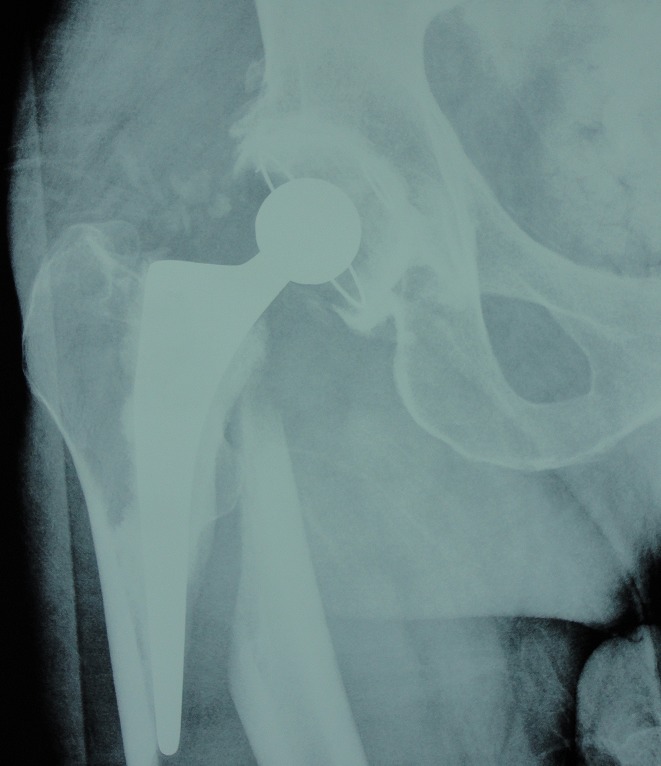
Radiographie de la hanche d'un patient qui présente une fracture sur prothèse totale de la hanche classée stade B selon la classification de Vancouver
